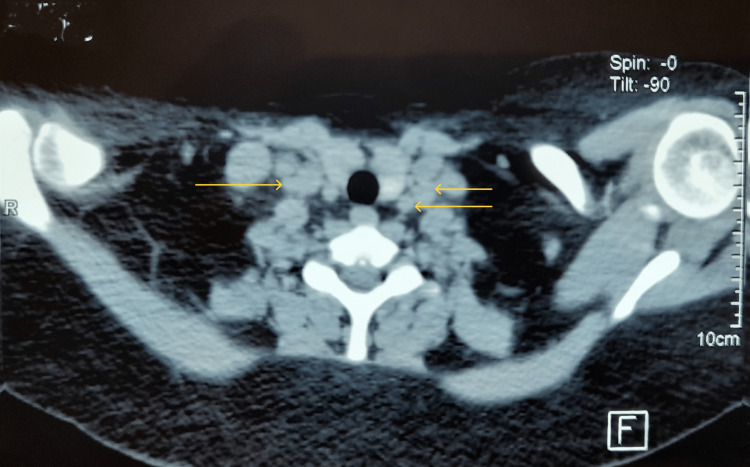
Thoracic CT showing the mediastinal adenopathies (arrows)
